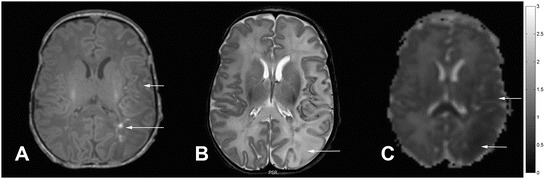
MRI scan taken at 3T on day 5. (a) T1 weighted axial image demonstrating generalised low signal
